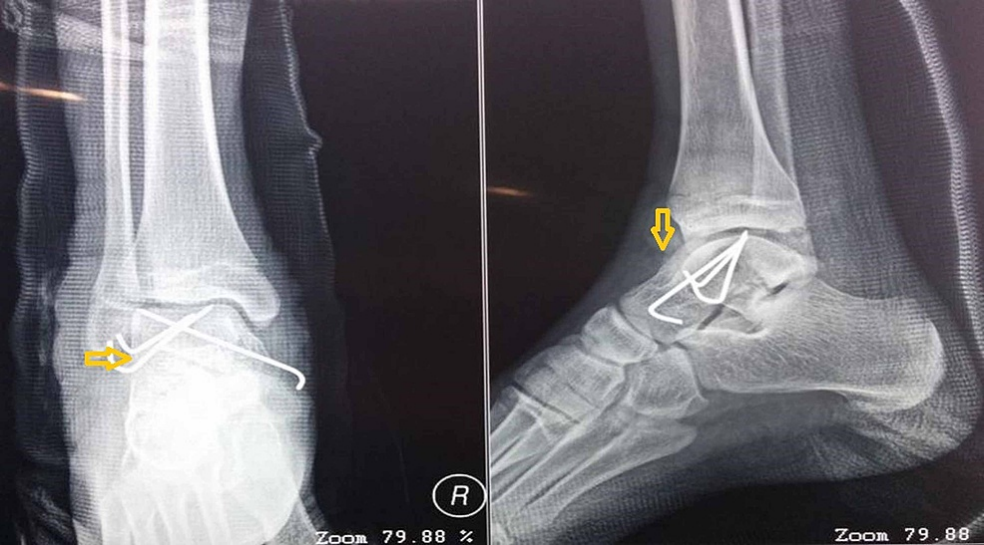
Post operative follow-up radiographs
